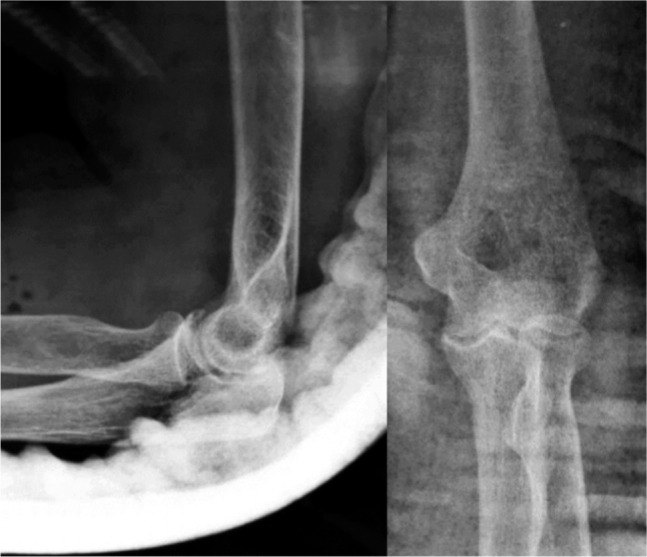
Post-reduction elbow radiographs (Lateral and AP) showing concentric reduction of the left elbow joint (upper limb in backslab).
